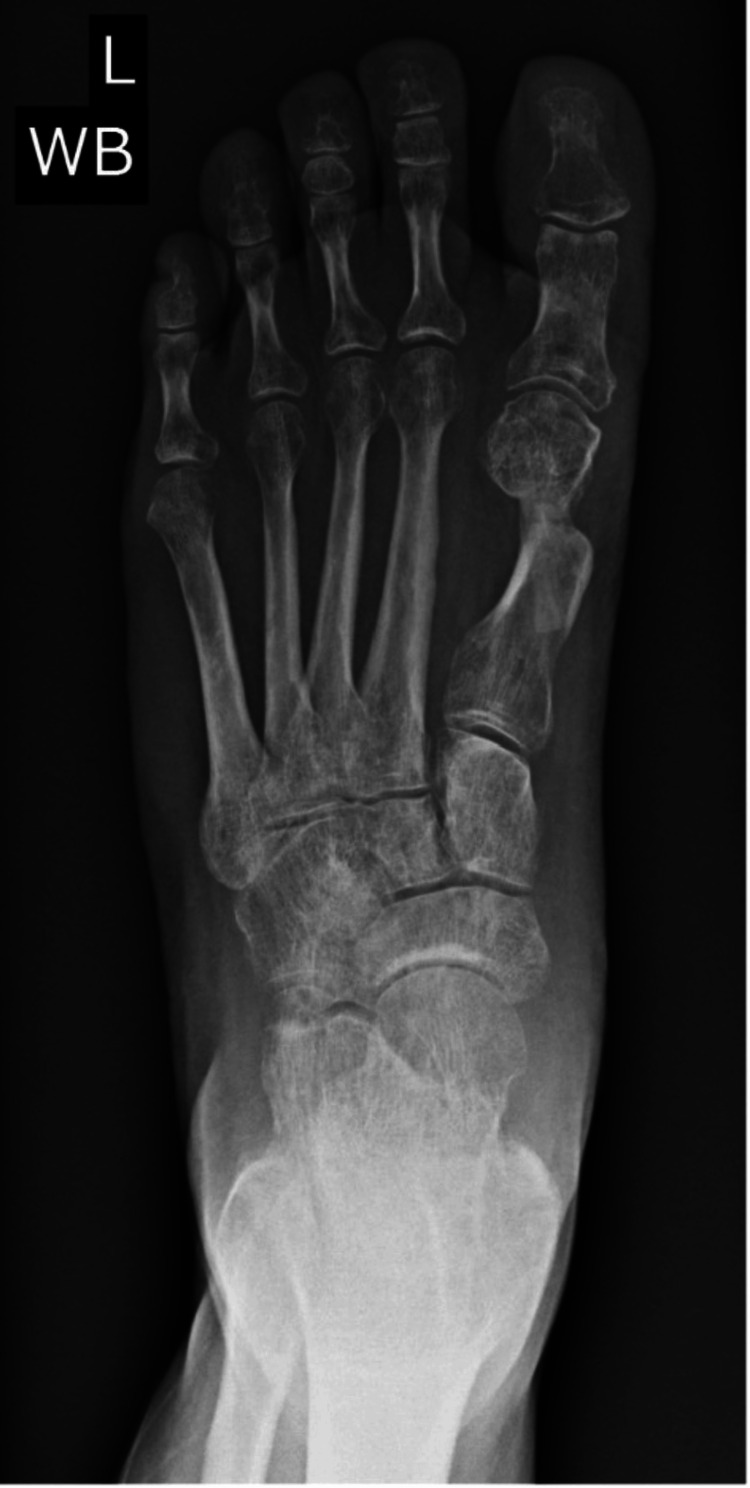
Radiograph three months after revision surgery showing robust callus formation, decreased radiolucency, and progressive osseous union
